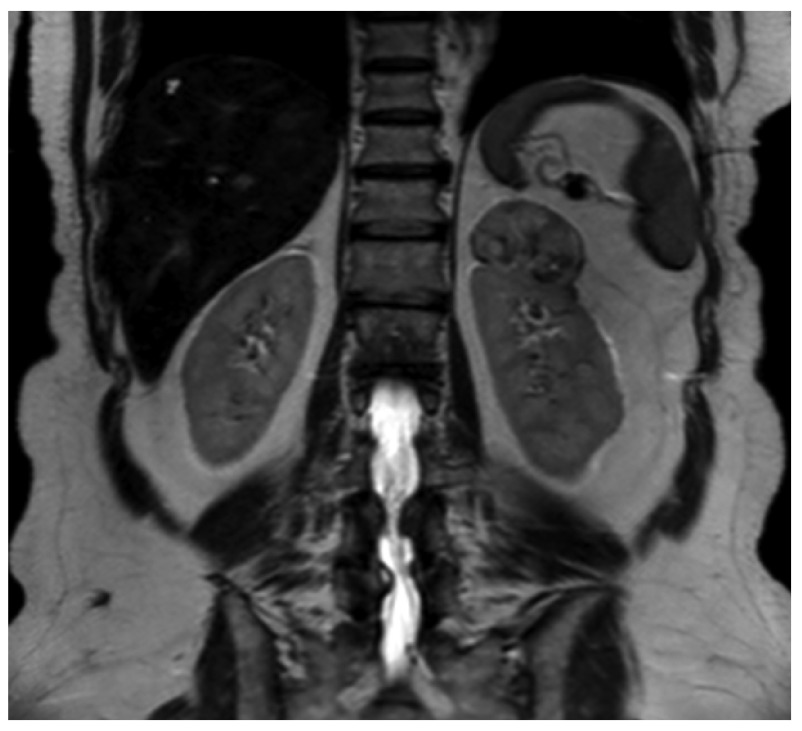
Mass in the left upper renal pole (T2-weighted MR image) with a size of 48×48×33 mm having a diffusely heterogeneous structure.
